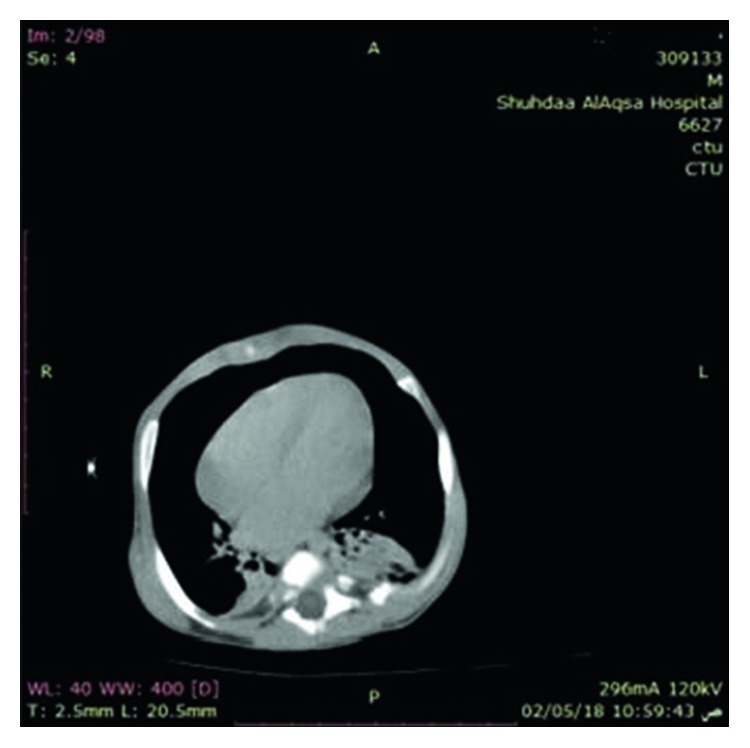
Chest CT showed the patchy area of consolidation in the posterior segment of the lower lobe of the lung.
